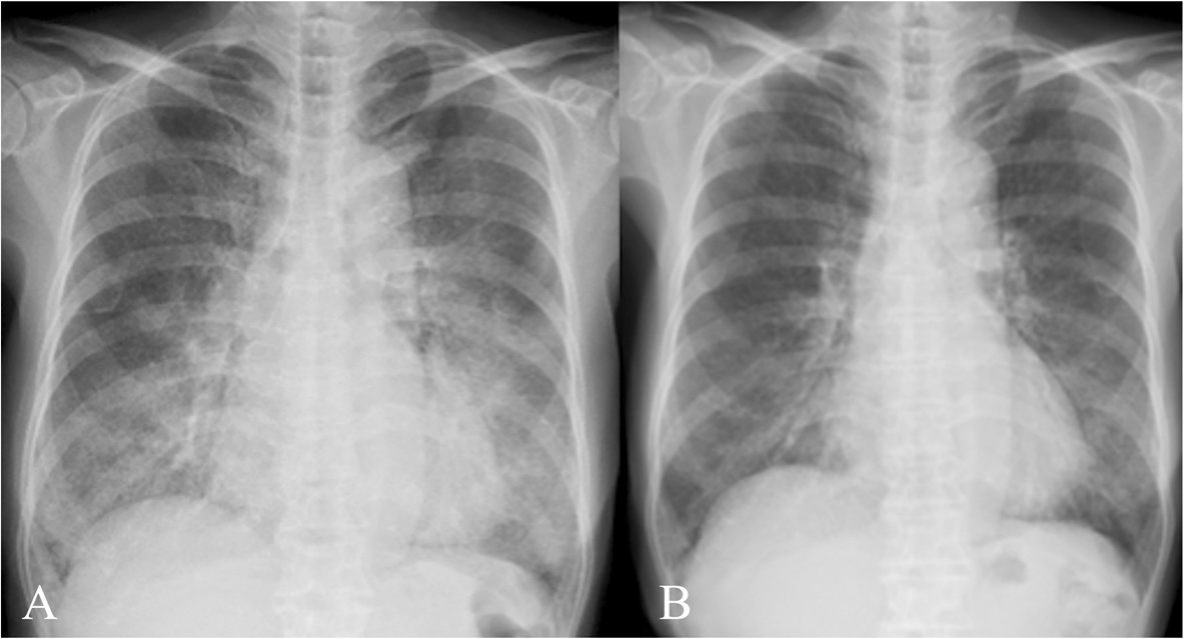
Chest radiographs before and after the treatment. (A) Chest radiographs (CXR) on admission. (B) CXR findings improved after one month of treatment with ambroxol.
